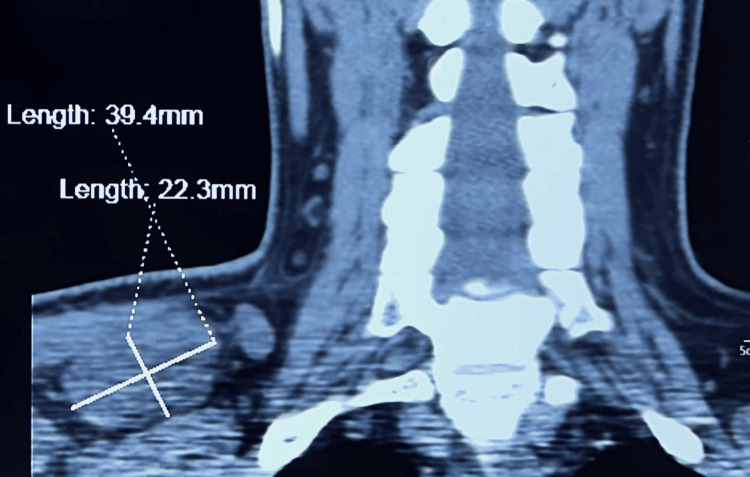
Cervical-thoracic CT scan performed 36 months after treatment revealing a right supraclavicular mass.
